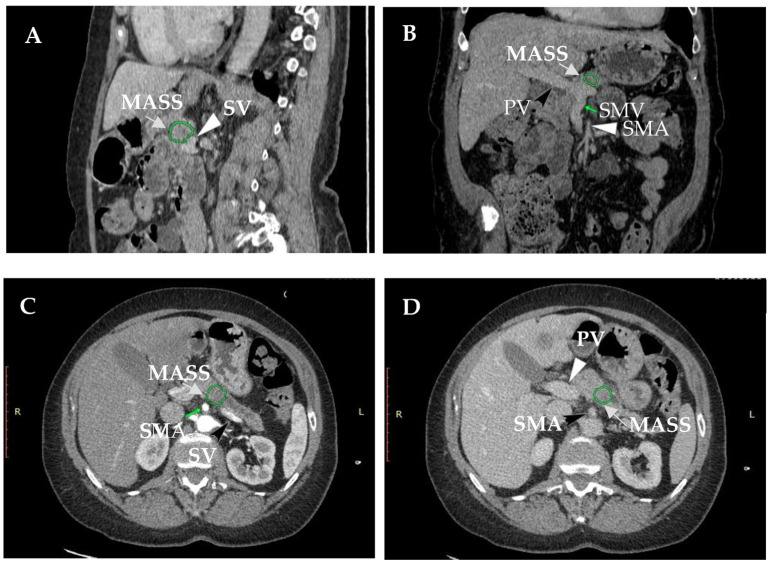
CT image of resectable pancreatic ductal adenocarcinoma (R-PDAC). (A,B) A coronal and (C,D) and axial base during the pancreatic phase of CT demonstrating hypovascular mass in pancreatic body without PV, SMA and SV involvement. SMV—superior mesenteric vein, PV—portal vein, SMA—superior mesenteric artery, CA—celiac artery, CHA—common hepatic artery, PHA—proper hepatic artery, SV—splenic vein.
